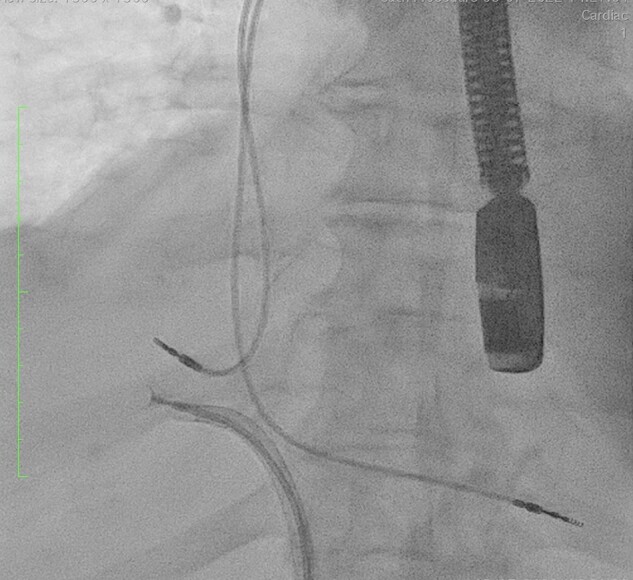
Transoesophageal echocardiogram and fluoroscopy-guided endomyocardial biopsy of cardiac mass. A steerable Agilis catheter is used to position the bioptome at the anterior right atrial wall to obtain tissue sample.
